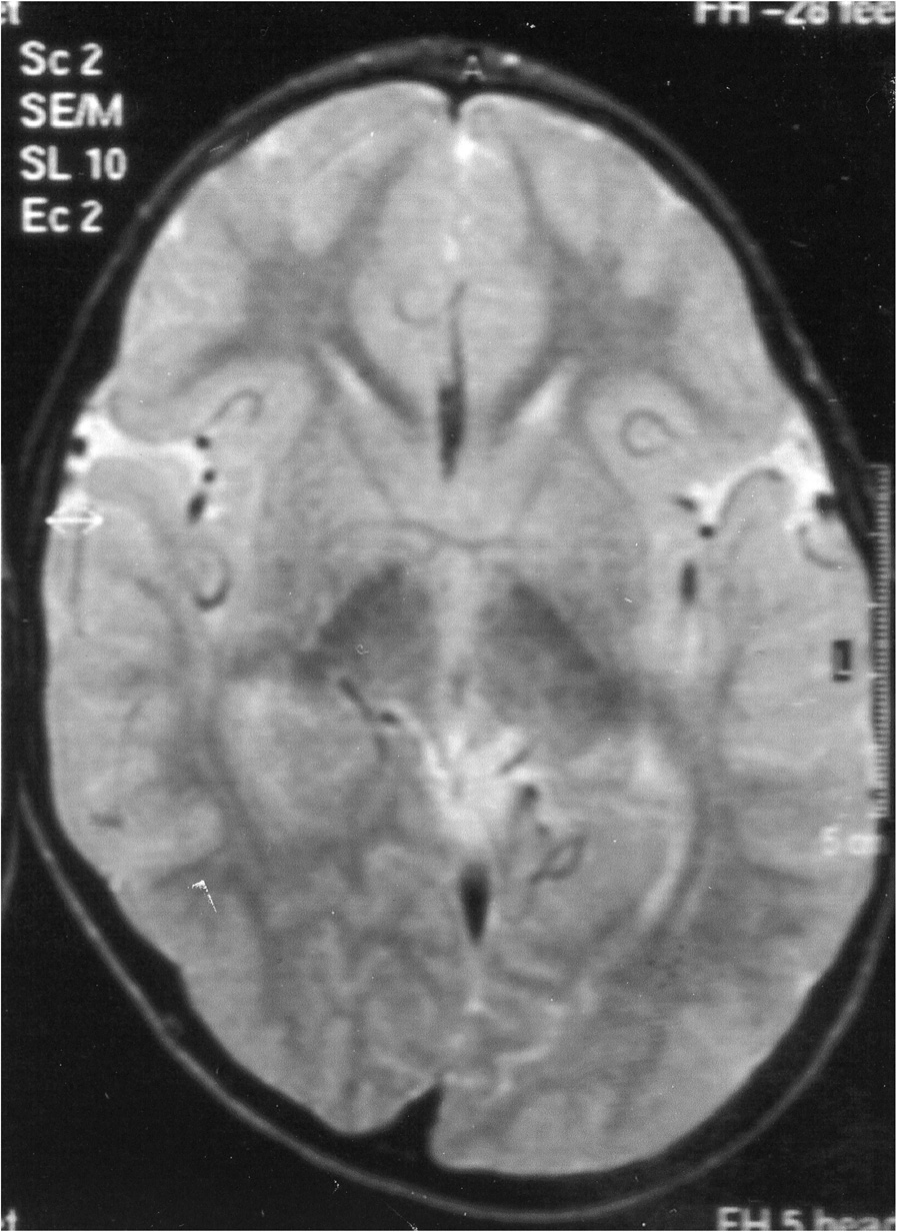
Axial PD-weighted magnetic resonance imaging of the Brain, performed at 3 years of age, shows hemispheric asymmetry with size increase of left posterior hemisphere.
